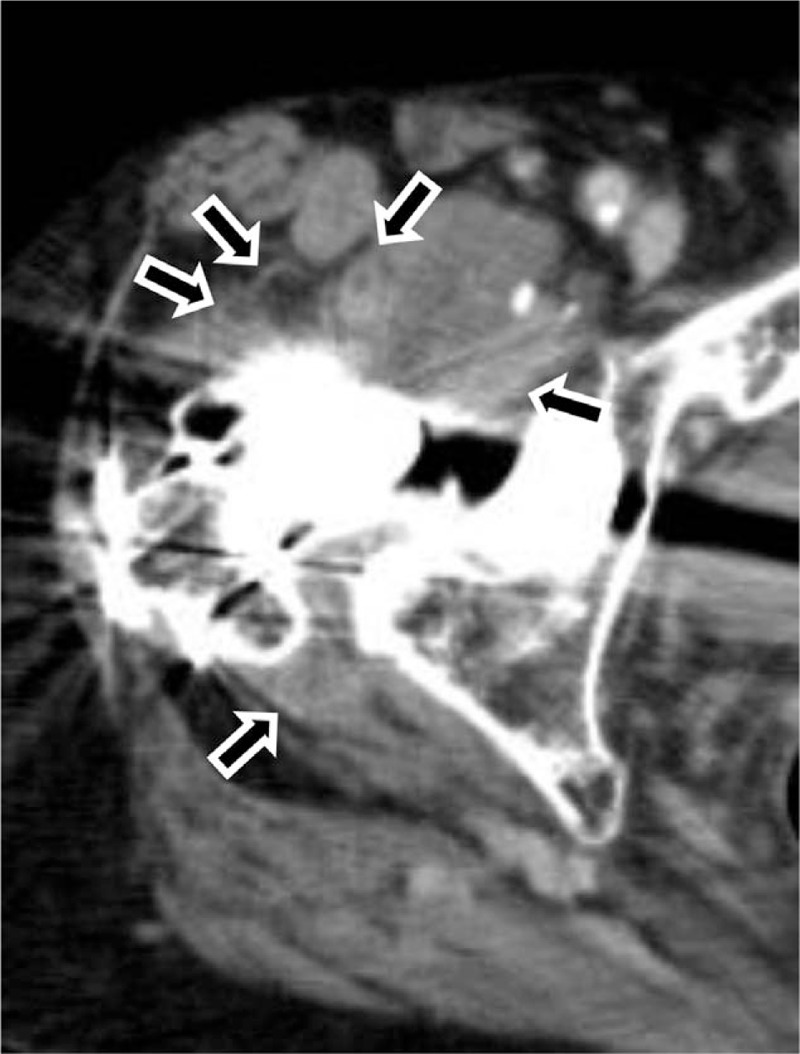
Radiological images. Contrast-enhanced computed tomography scan showing a ring-enhancing region around the right hip joint suggesting abscess formation (black arrow).
